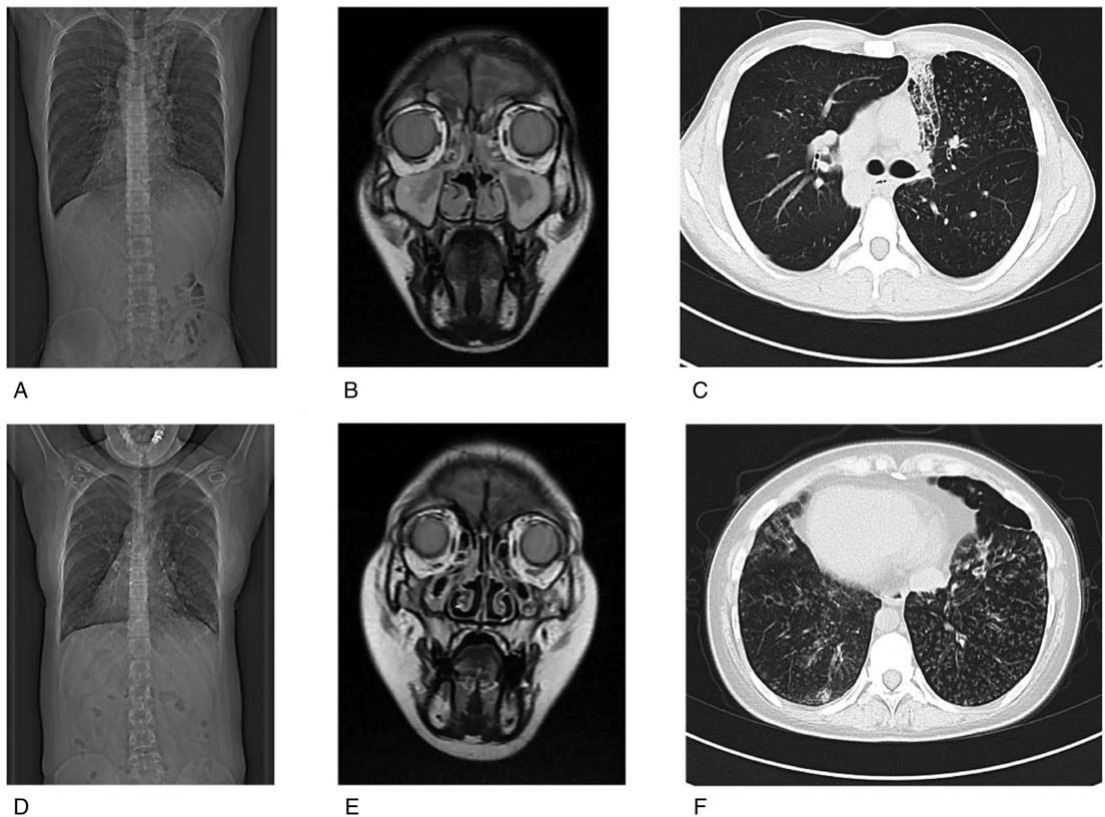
The radiological examination of Proband (IIII-5), (A) CT showed situs inversus. (B) MRI of paranasal sinus shows bilateral ethmoid sinusitis and maxillary sinusitis. (C) HRCT of chest shows bronchitis and bronchiolitis. The radiological examination of Proband's sister (III-1), (D–F) the same but more severe imaging appearance as the proband. CT = computed tomography, HRCT = high-resolution computed tomography, MRI = magnetic resonance imaging.
